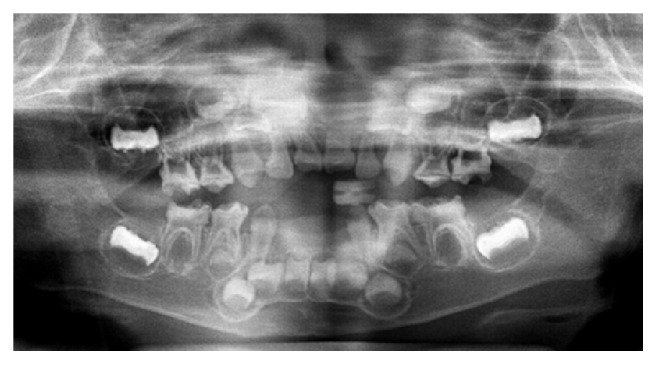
Panoramic radiographic appearance at age of 3 years and 2 months.
